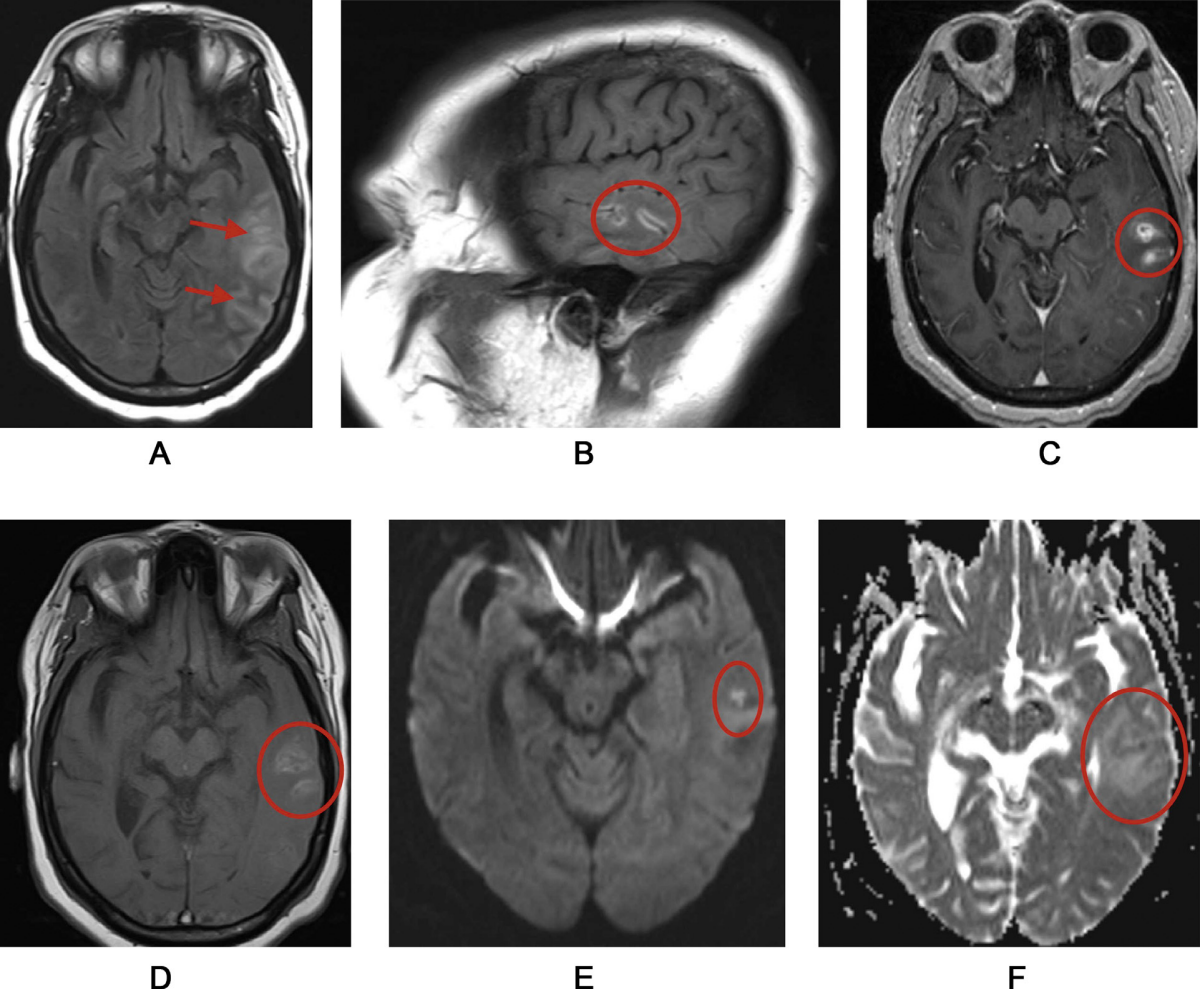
MRI reveals mildly expansile T2 FLAIR hyperintensity in the left temporal lobe, with cortical T1 hyperintensity, nodular leptomeningeal enhancement, and small foci of restricted diffusion. These indicate active inflammation, vasogenic edema, suspected cortical laminar necrosis, and cytotoxic edema of Neurosarcoidosis with associated vasculitis affecting the left temporal lobe.
